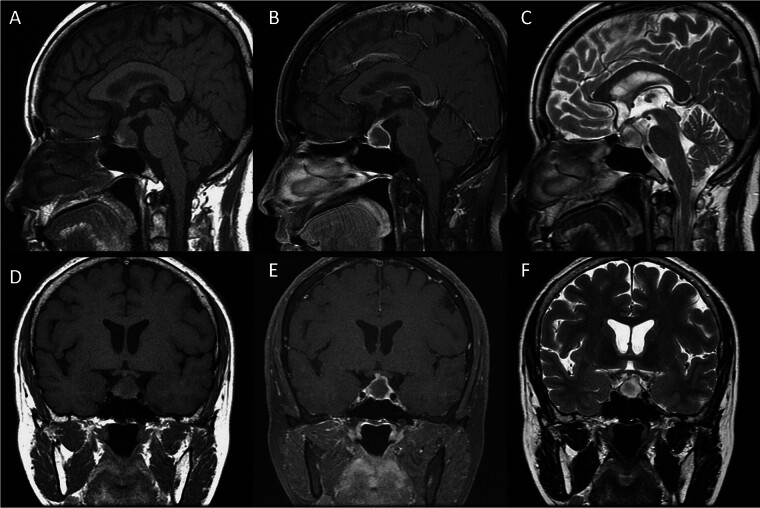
Preoperative brain MRI. Sagittal T1WI (
